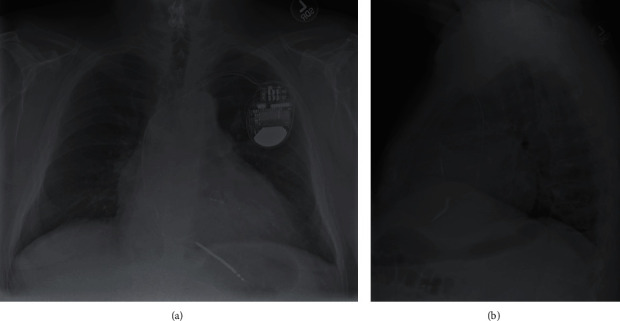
A two-view chest X-ray demonstrating enlarged cardiac silhouette, minimal bibasilar atelectasis, and appropriate placement of pacemaker leads (a, b).
